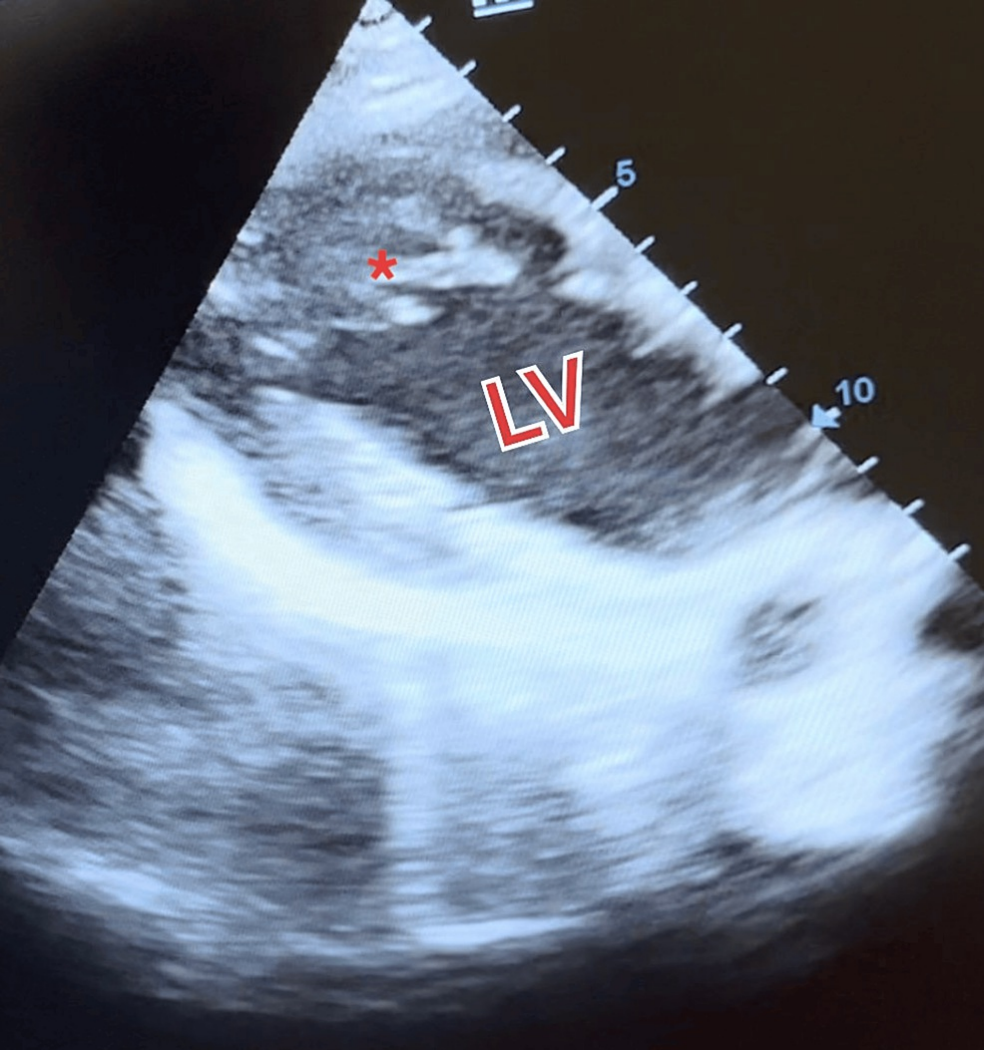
Transthoracic echocardiogram demonstrating left ventricular non-compacted myocardium and trabeculations (red asterisks)
